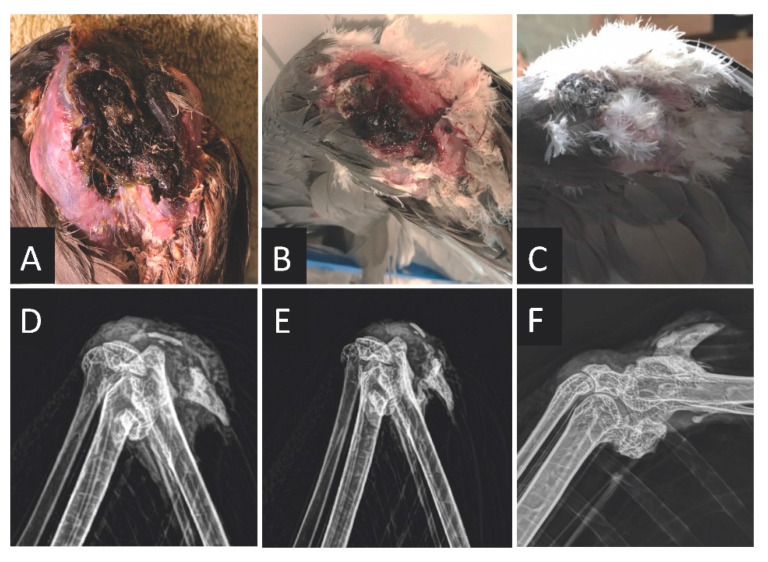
The left carpus of Case 3, an 11-year-old male wattled crane (*Bugeranus carunculatus*), with septic arthritis and suspected alular osteomyelitis at the time of presentation and after treatment with intravenous antibiotic regional limb perfusion. Palmodorsal (PD) photographs of the wound (A) 4 days after presentation, (B) 6 weeks later, and (C) 10 weeks after presentation. PD radiographs (D) at the time of presentation, (E) 6 weeks later, and (F) 16 months after presentation.
